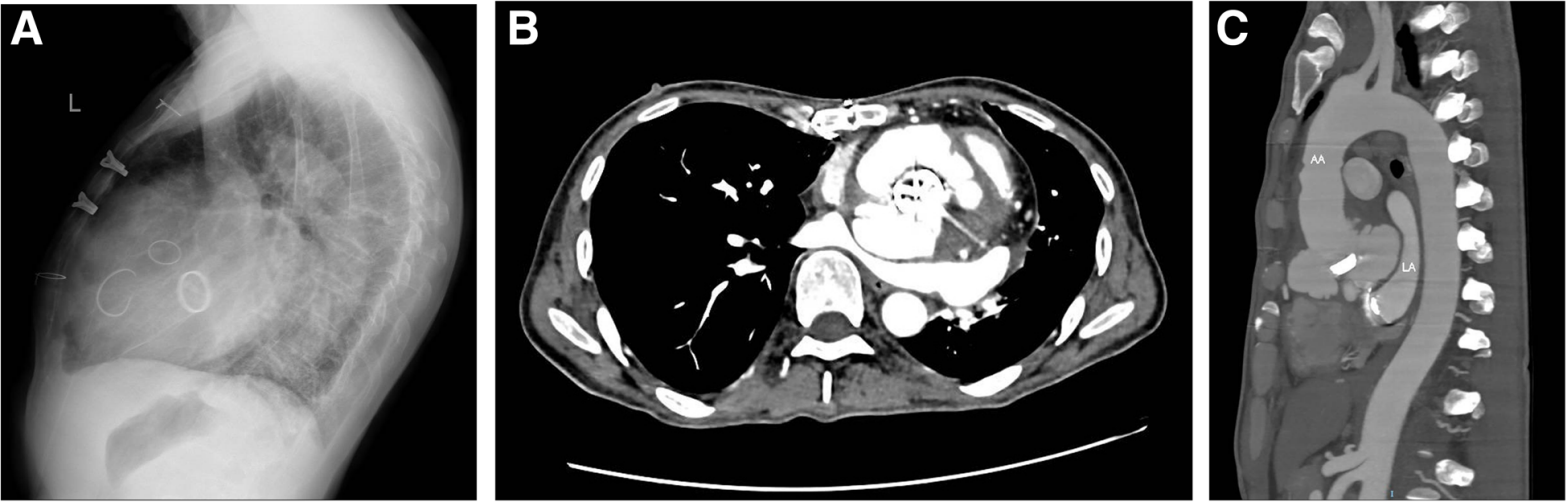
Lateral chest X-ray (A) indicates that the right ventricle is closely attached to the sternum, axial CT image of the chest (B) indicates that the distance between the aortic root pseudoaneurysm and the sternum is small, and sagittal CT image (C) shows the ascending aorta is closely attached to the sternum
